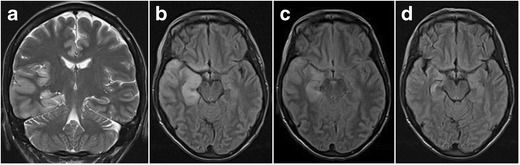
Status epilepticus. A 30-year-old woman with epilepsy quit her anti-epileptic treatment during pregnancy and was brought to the hospital in status epilepticus. Coronal T2W (a) and axial FLAIR images (b) in the acute phase show extensive cortical oedema in the right temporal lobe with involvement of the right hippocampus as well as in the right insula. There was no diffusion restriction or contrast enhancement (images not shown). Control MRI performed 4 days later after initiation of anti-epileptic treatment shows regression of the oedema on axial FLAIR (c). On follow-up MRI performed one month later, axial FLAIR shows volume loss of the right hippocampus as well as increased signal intensity, reflecting secondary gliotic changes (d). Alternatively, this could also be a preexistent mesial temporal sclerosis. As extensive clinical work-up revealed no other possible cause for the observed cortical oedema, final diagnosis was seizure-induced cortical edema
